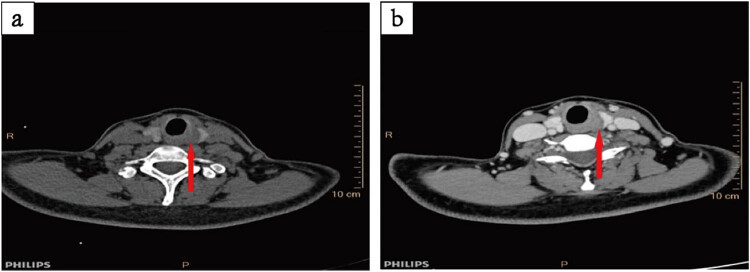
Preoperative soft tissue axial CT scan of the neck (a) + enhancement (b): the left lobe of the thyroid gland shows a hypodense nodule with blurred margins (about 0.6 cm in diameter).
